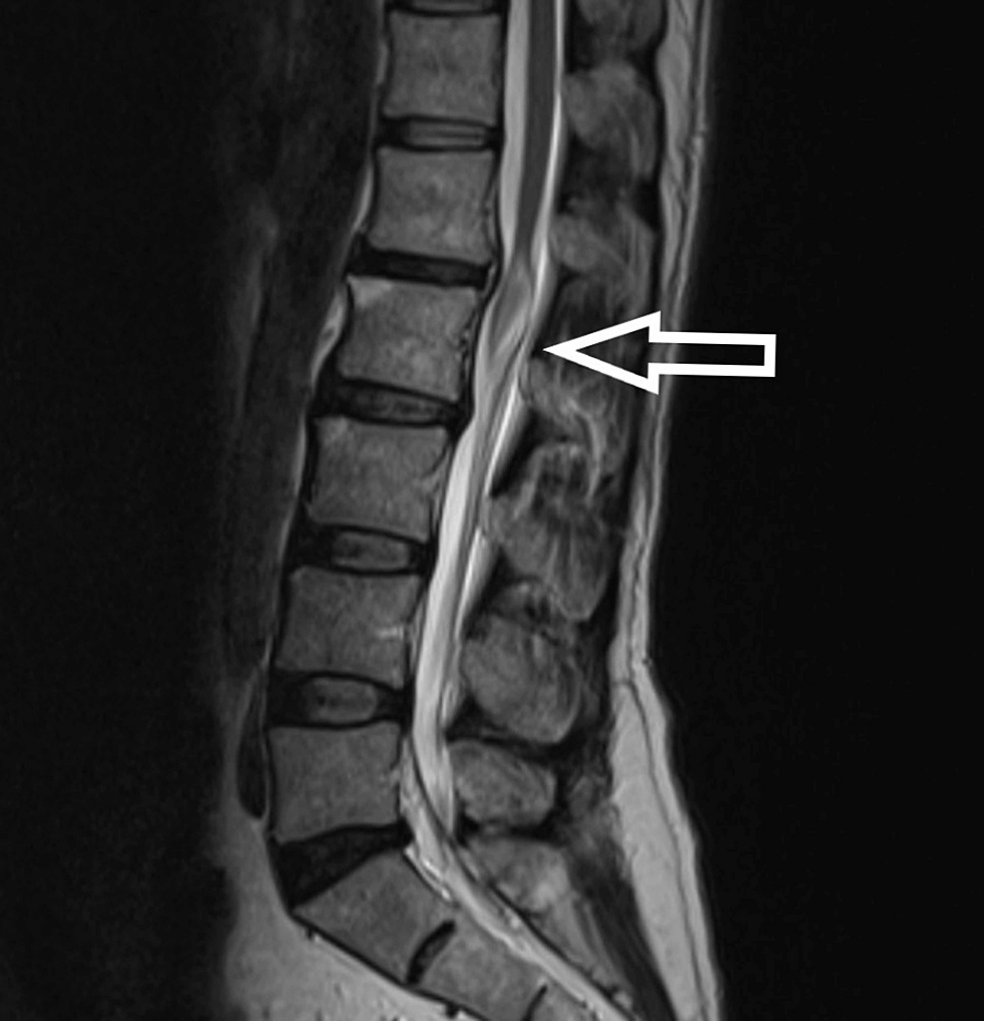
T2-weighted MRI (sagittal) showing hyperintense intramedullary mass
